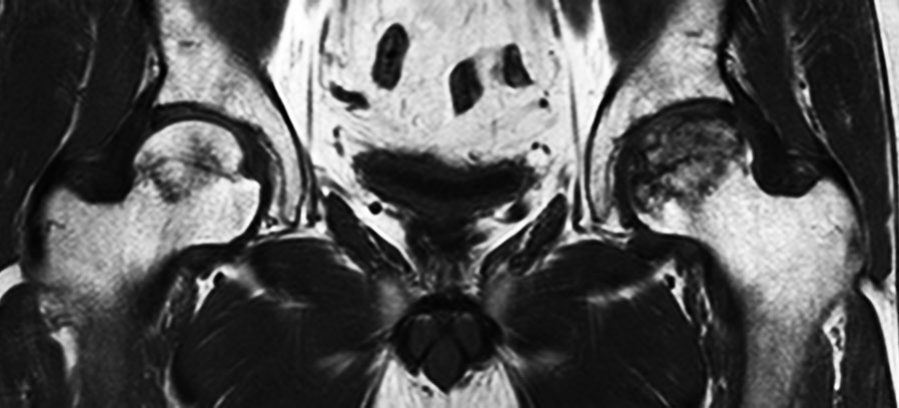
A coronal T1-weighted image obtained 10 months after the initiation of corticosteroid therapy (6 weeks after the first examination). Note the new T1 low-intensity band in the right femoral head
